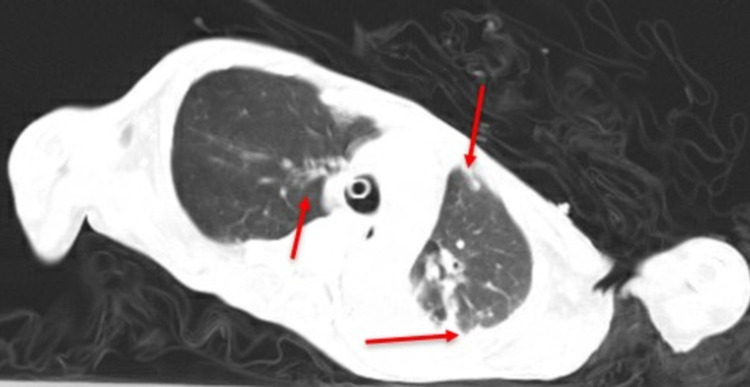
A CT scan of the chest taken during this admission showing bilateral areas of patchy airspaces and infiltrates (red arrows)
